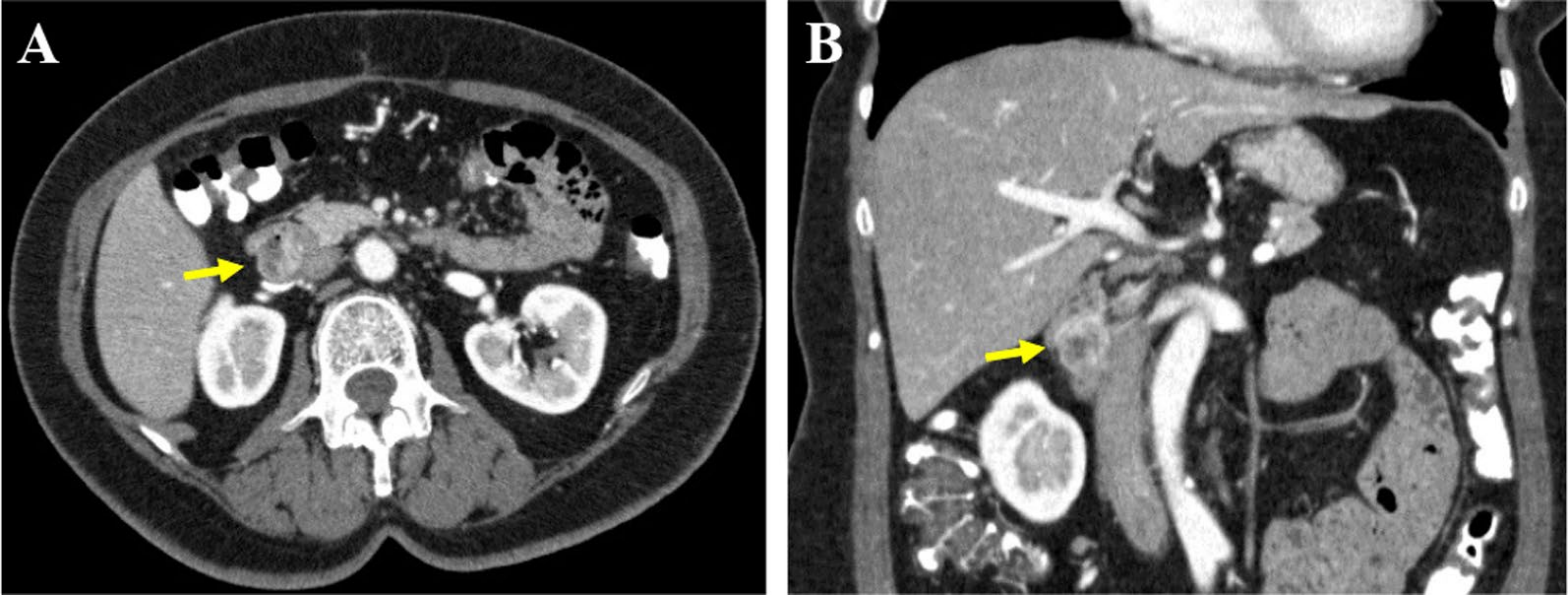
A, B Contrast-enhanced computed tomography at the first visit. Extravasation was seen from a 20-mm mass in the second part of the duodenum (arrow)
